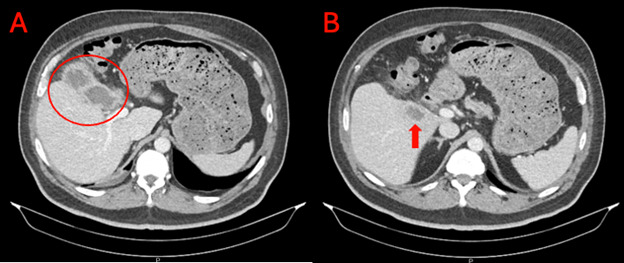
Representative image from a CT scan. (A) A thickened, irregular gallbladder wall with pericholecystic fat stranding representing acute gangrenous cholecystitis. (B) A 2.7-cm rim-enhancing liver hypodensity suggestive of an abscess.
